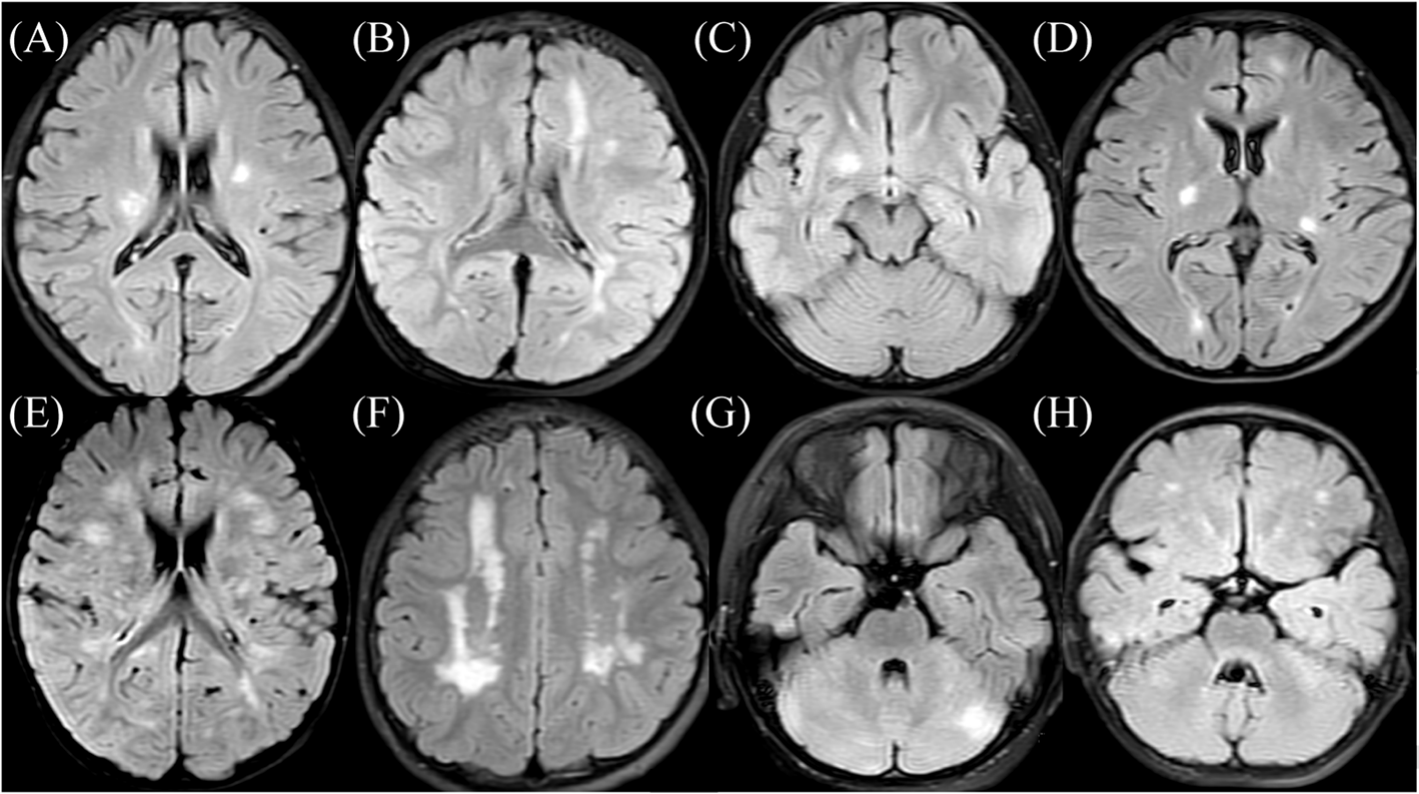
Brain MRI findings of patients with MOG-Ab-negative ADS (A–H)
